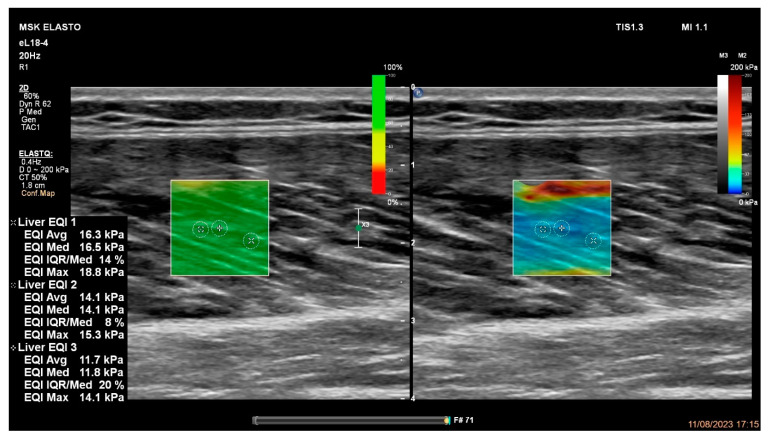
An example of the semimembranosus muscle stiffness measurement using ultrasound-based shear-wave elastography.
